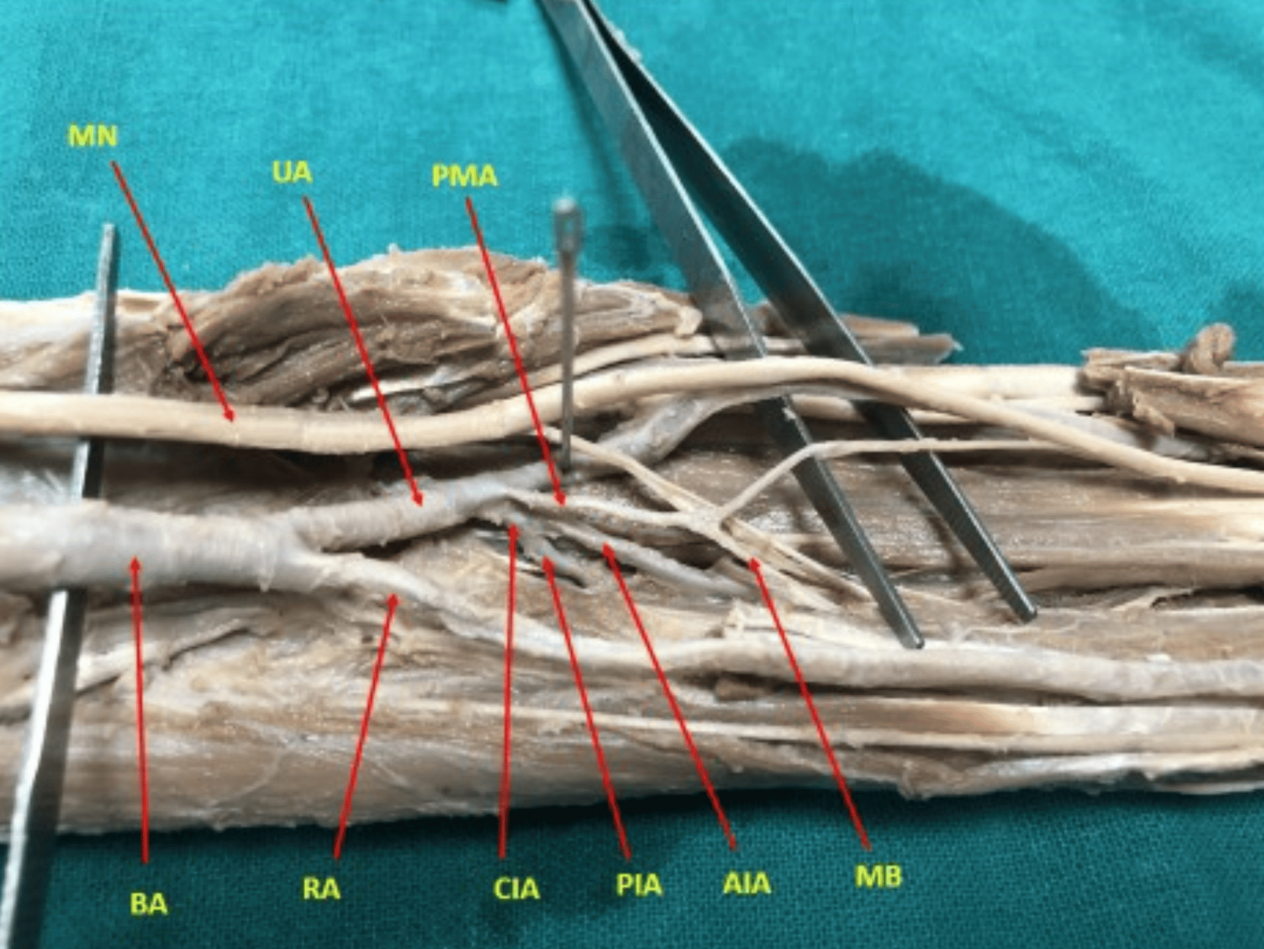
Antebrachial type of PMA blending with the perineurium of the median nerve in the proximal forearm BA: brachial artery, UA: ulnar artery, RA: radial artery, MN: median nerve, PMA: persistent median artery, CIA: common interosseous artery, AIA: anterior iterosseous artery, PIA: posterior interosseous artery, MB: muscular branch, CT: carpal tunnel
